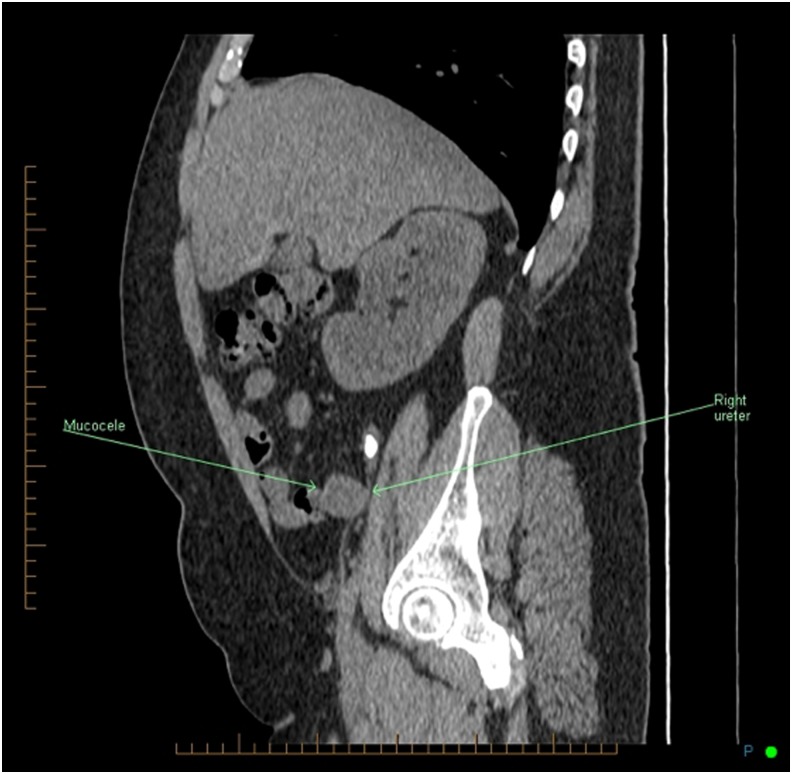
Initial sagittal noncontrast CT scan image showing an appendiceal mucocele compressing the right ureter below the midureteral stone.
